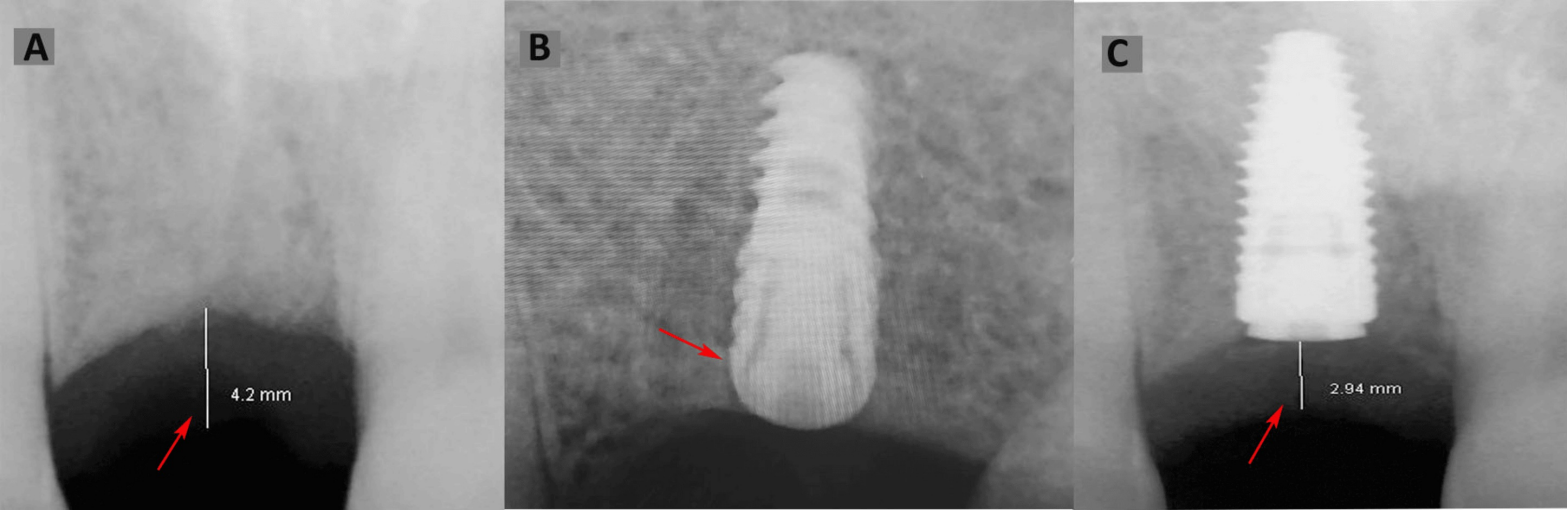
Estimation of crestal mucosal thickness. (A) Preoperative crestal mucosal thickness (in mm) as measured on CBCT;
